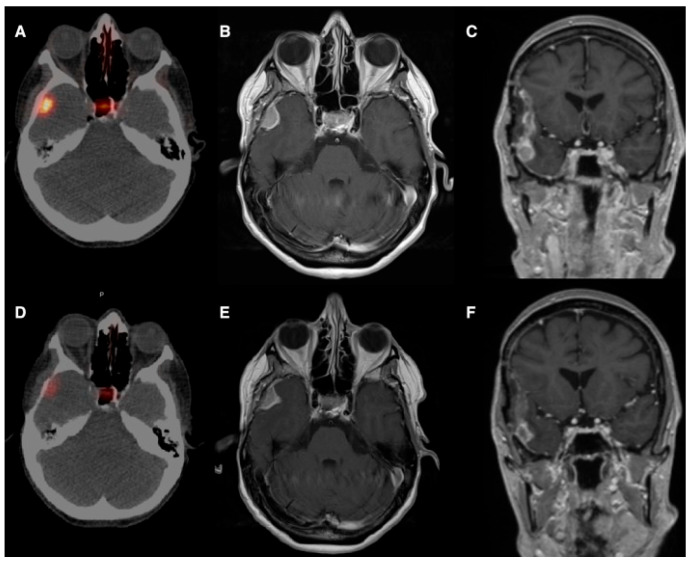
(A). Baseline ^68^Ga-DOTATOC PET/CT shows high SSTR expression in the right-temporal meningioma and normal pituitary uptake. (B,C). Gadolinium-enhanced T1 MRI shows a uniformly enhancing solid lesion. (D). Post-treatment gallium-68-DOTATOC PET/CT shows a 79% decrease in SSTR2 expression. (E,F). Post-treatment T1 MRI shows a partial response with a 38% volume and 24% diameter reduction, central necrosis, and reduced enhancement in the prior resection cavity. Reprinted from [19] under a creative commons attribution 4.0 international license (http://creativecommons.org/licenses/by/4.0/, accessed on 31 October 2025). No changes were made.
